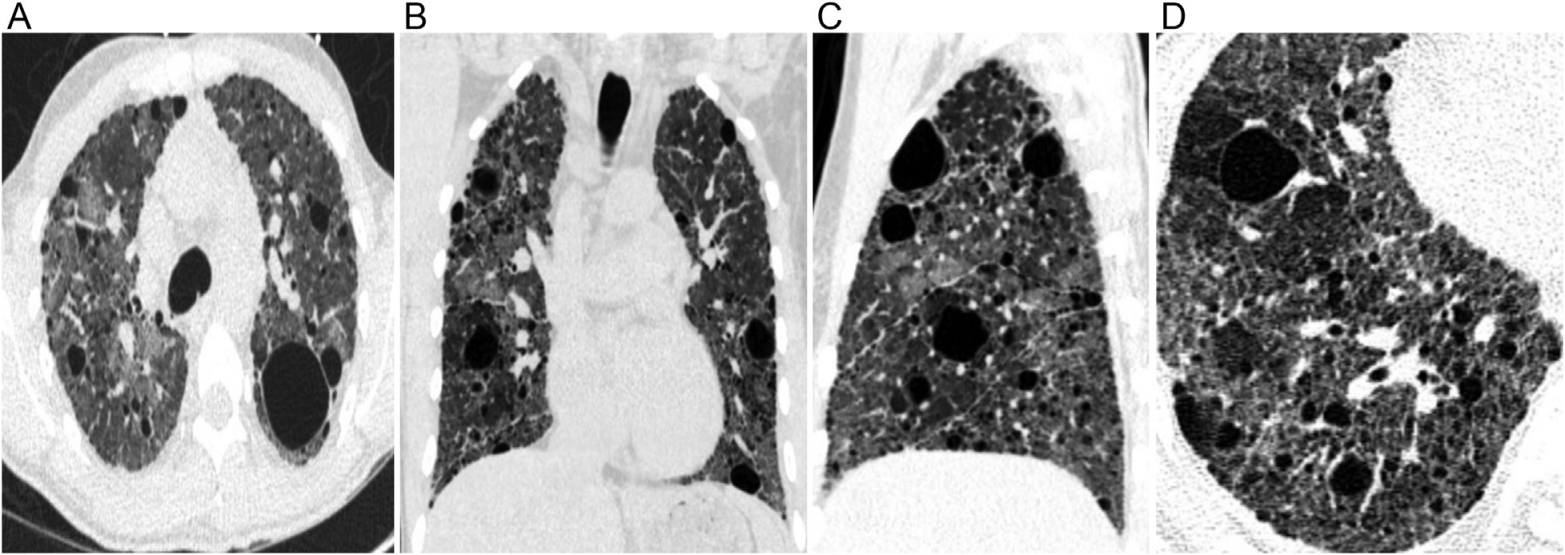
Imaging findings at 27 years of age (case 1). Axial (A) and coronal (B) noncontrast-enhanced chest CT slices demonstrate patchy ground glass opacities, reticulations, interlobular septal thickening, and multiple variable size thin-walled cysts throughout the lungs. A sagittal image through the right lung (C) demonstrates the diffuse distribution of the findings. Small field of view axial image (D) demonstrates subpleural and central reticulations with thickening of bronchovascular bundles consistent with pulmonary fibrosis.
